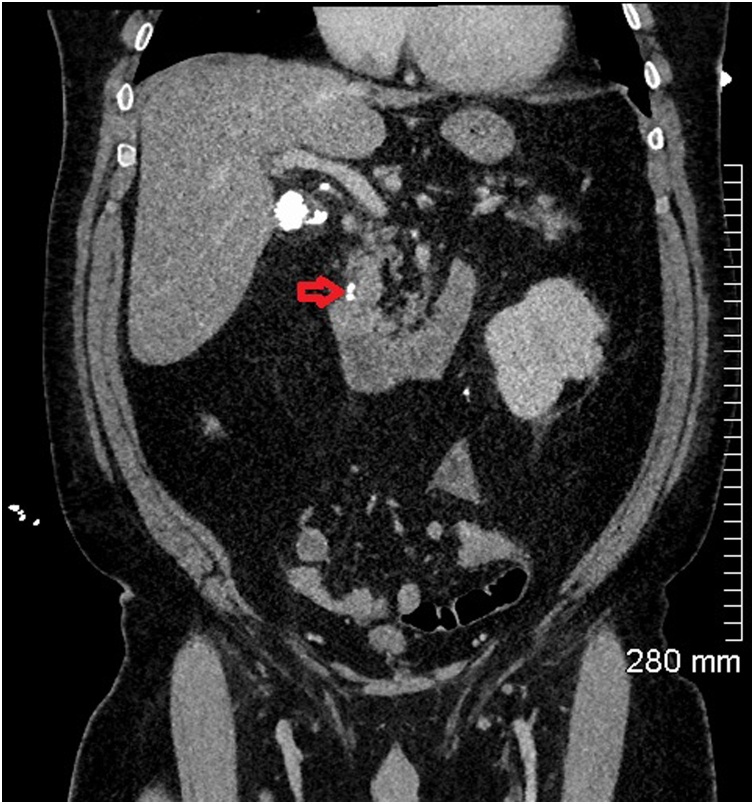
Coronal CT abdomen showing more calcified gallstones in the distal common bile duct (red arrow).
